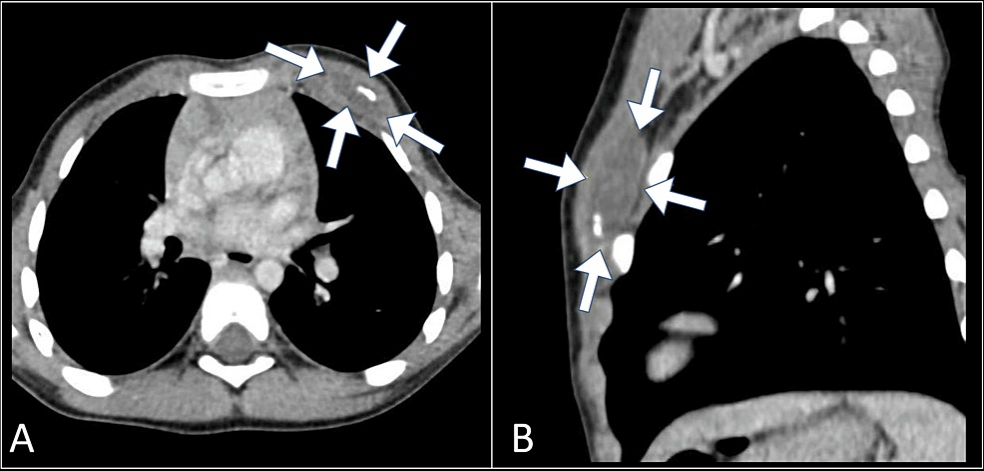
Axial (A) and sagittal (B) CT images revealing a collection (arrows) within the left chest wall CT: computed tomography
